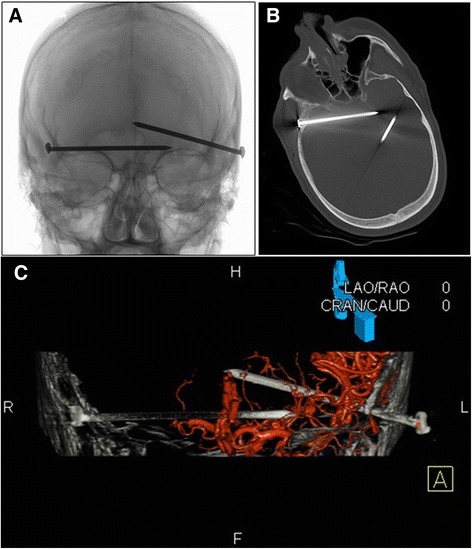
a Skull X-ray. b Computed tomography head – bone window showing two nails penetrating the calvarium on each side. There was evidence of subarachnoid hemorrhage on computed tomography. c Cerebral angiogram three-dimensional reconstruction. Three-dimensional rotations re-demonstrated the presence of bilateral temporal horizontal metallic nails coursing adjacent to the main proximal intracranial vessels. No definite contrast extravasation, arterial occlusion, stenosis or pseudoaneurysm was identified
